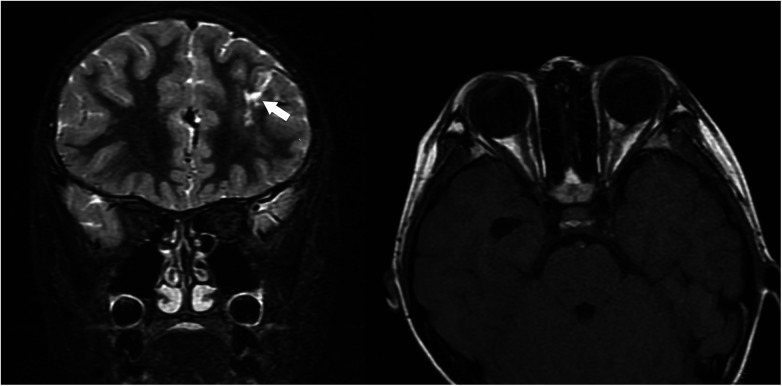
One month after anthelmintic treatment, orbital MRI of the elder twin sister demonstrated marked improvement in left periorbital soft tissue swelling and reduced extent of the left frontal lobe patchy shadow compared with prior imaging. (Left: Coronal T2-weighted orbital MRI, lesion indicated by the white arrow; Right: Axial T1-weighted orbital MRI).
